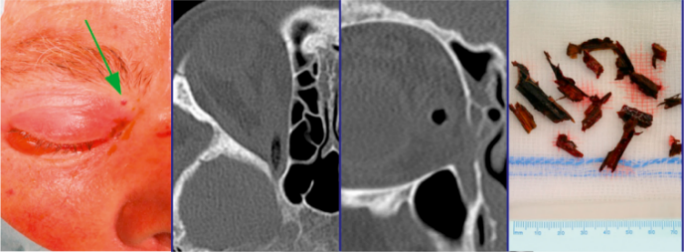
Foreign body of the orbit. In the CT scan the biological material is detected as gap. The foreign body caused a minimal wound when penetrating (arrow in the left image). It was stopped in the orbital tip. Removal via an endonasal approach.
